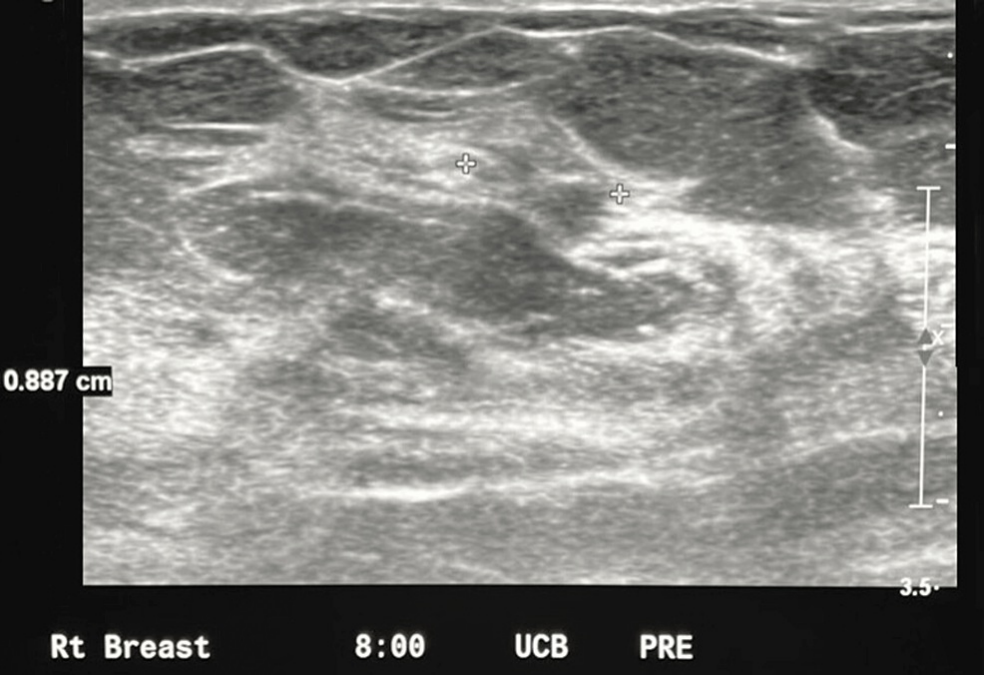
Ultrasound of the right breast. The 0.9 cm mass in the 8:00 position.
